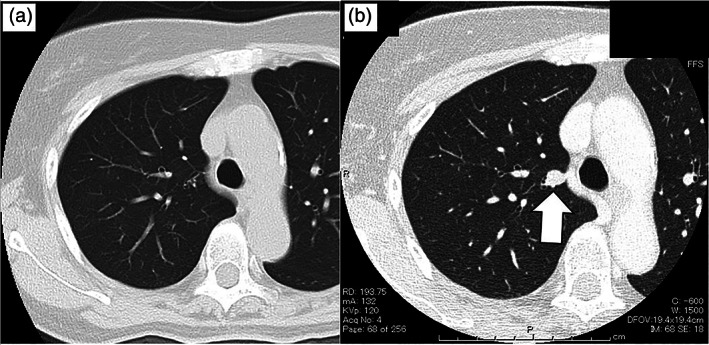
(a) Chest computed tomography (CT) image 4 months after the initial examination revealed no apparent nodule. (b) CT findings 7 months later (a) revealed a 1 cm diameter pulmonary nodule on the right side of the azygos vein (white arrow).
